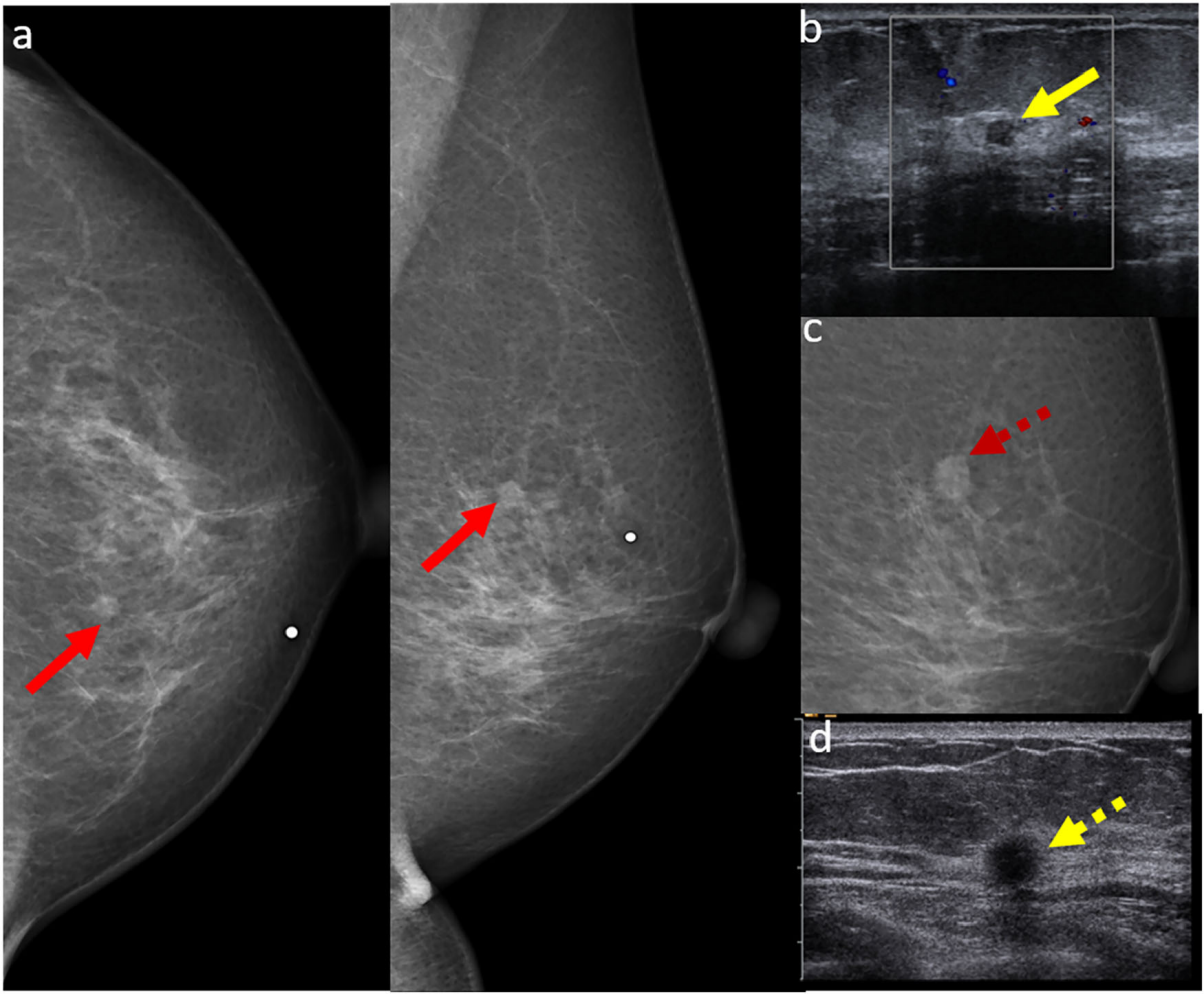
a A 56-year-old woman underwent baseline screening mammography. CC and MLO left mammograms demonstrated a circumscribed isodense mass with indistinct margins in the left breast (red arrows). b Workup ultrasound described an anechoic circumscribed subcentimeter mass (yellow arrow) corresponding to the mammographic finding interpreted as a cyst. In light of the patient’s age, it was categorized as BI-RADS 3, and a short-term follow-up was recommended. c Six-month follow-up MLO mammogram (CC view not shown) showed an interval increase in size of the mass with irregular margins (red dotted arrow). d Six-month follow-up ultrasound demonstrated an irregular hypoechoic mass with microlobulated margins and posterior shadowing (dotted yellow arrow), BI-RADS 4, suspicious for malignancy. Ultrasound-guided core biopsy revealed grade 3 triple-negative breast cancer
